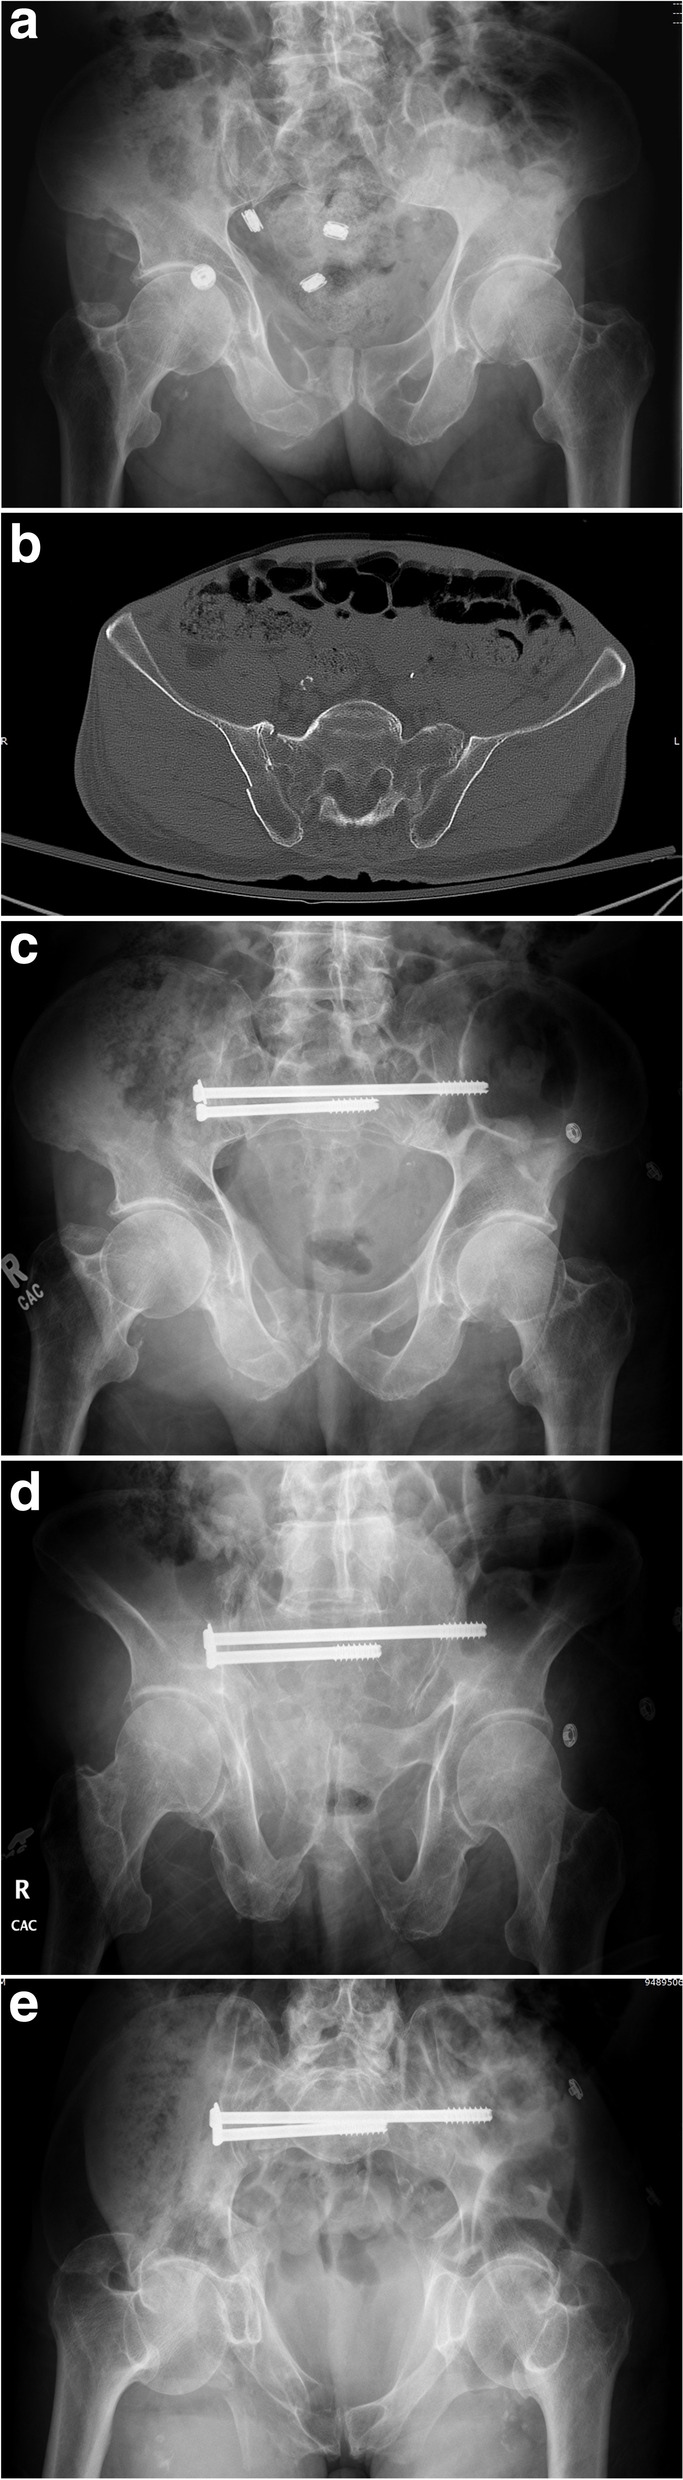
Radiographic and axial CT pelvic image of a right SI fracture-dislocation (A-B) treated with a right trans-sacral screw and right ilio-sacral screw to prevent implant failure and fracture re-displacement (C-E)
